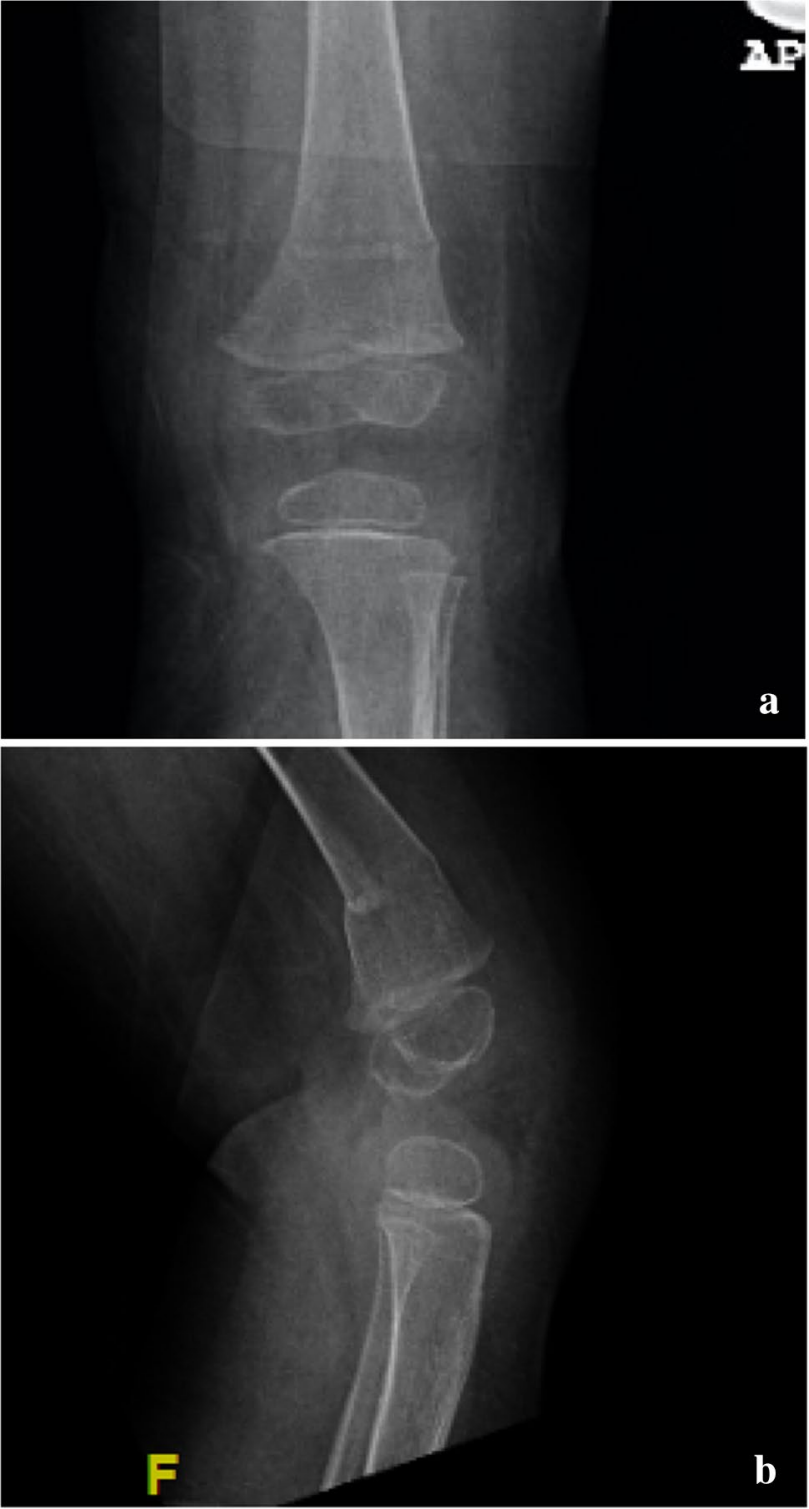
a Anteroposterior and b lateral femur radiographs showing osteoporotic fracture after casting, following ipsilateral open reduction
